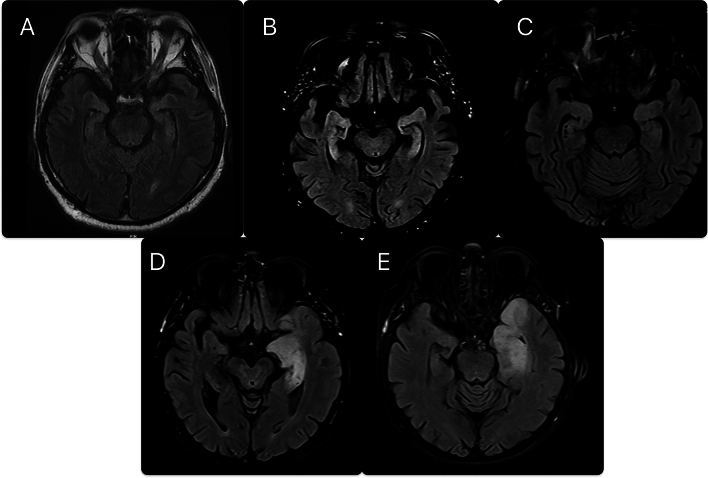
(A–C) from patients 1–3 respectively showing MRI FLAIR bilateral mesiotemporal lobe hypersignals;(D) Patient 5 initial MRI FLAIR sequence with left mesiotemporal hypersignal mistaken for autoimmune encephalitis; (E) Follow-up MRI of patient 5 showing expansion of the lesion beyond the limbic system and suggestive of neoplasm.
